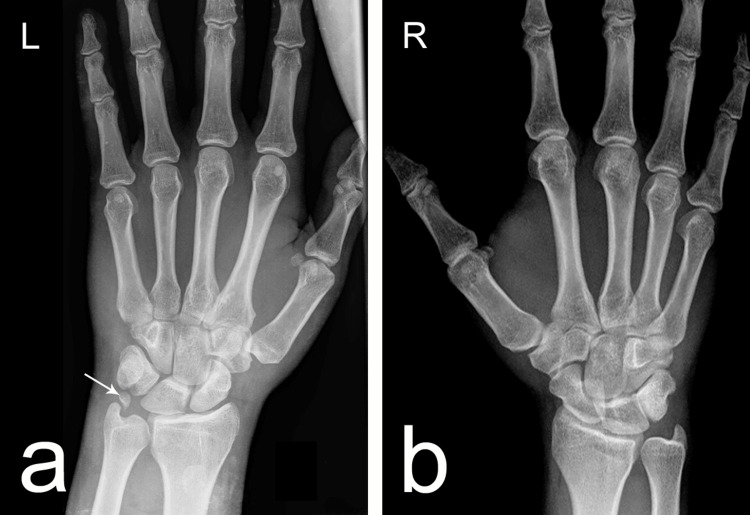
Anteroposterior radiographs of the wrists. (a) The left wrist demonstrates the presence of an os triquetrum secundarium (OTS), indicated by the white arrow. The ossicle appears as a discrete, ovoid structure located between the triquetrum and the ulnar styloid process, with smooth cortical margins, a preserved cortical-to-medullary ratio, and a distinct radiolucent gap from adjacent bones—features consistent with a developmental accessory ossicle. (b) The right wrist shows typical carpal anatomy without accessory ossicles or osseous abnormalities.
